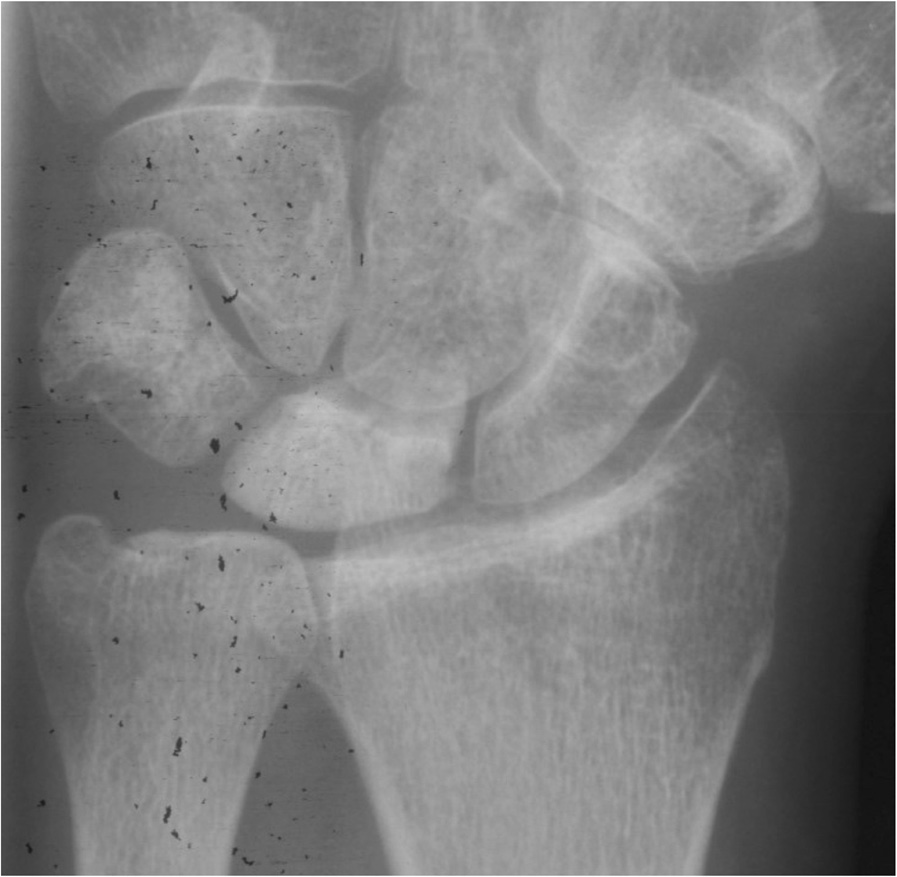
Pre-operative x-rays of the left wrist of of a 44-year-old male patient who had undergone VBG
